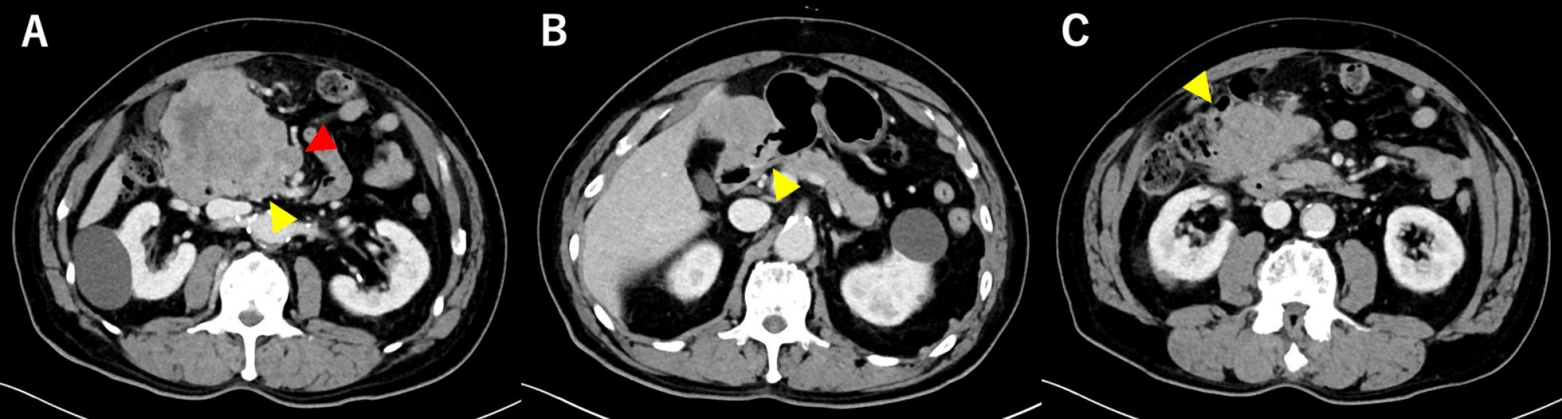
Contrast-enhanced computed tomography scan revealed a huge mass clumped with the swollen infrapyloric lymph node, invasion to the pancreatic head (A, yellow triangle), thick gastric wall with contrast effect (B), invasion to the descending part of the duodenum and transverse colon (C), and a continuous shadow defect from the tumor located in the superior mesenteric vein (A, red triangle)
